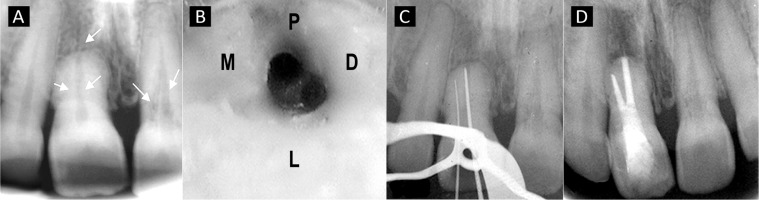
A) Preoperative radiograph showing the presence of additional canal in both 11 and 21; B)11Disto-labial position of additional canal to main canal under 3X magnification; C) Working length radiograph showing presence of 2 separate canals in; D) Post-obturation radiograph of 11
